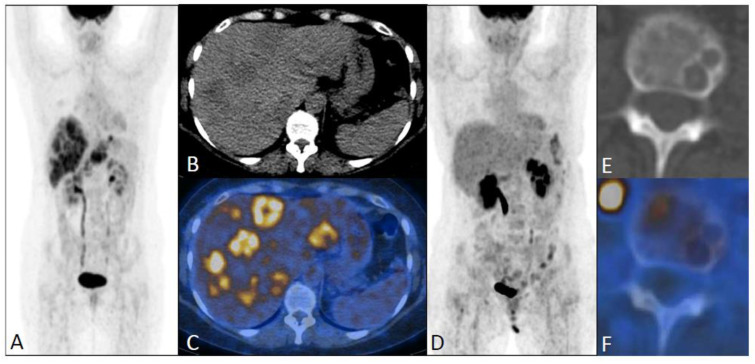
An FDG-PET/CT scan performed three years after the initial diagnosis of ILC (pT2N1, SBR II) in a 52-year-old patient treated by aromatase inhibitor, due to elevated tumor marker (CA 15.3 = 248). Maximum intensity projection (A), Axial CT (B), and PET/CT fusion (C) images showing metastatic spread to the liver, confirmed by biopsy. Maximum intensity projection (D) showed metabolic response after three cycles of chemotherapy. However, on this follow-up PET/CT scan, lytic bone metastases with no FDG-avidity were found, as shown on axial CT (E) and PET/CT fusion (F) images. This patient died 18 months after the diagnosis of recurrence.
